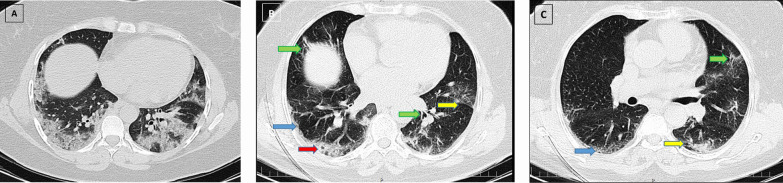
A 47-year-old female patient presented with fever and cough, who was admitted to hospital and was discharged on day 16 of admission. Ground glass opacities and consolidations in multiple pulmonary lobes are observed in the initial CT scan (A). The total CT severity score was calculated as 18, and the disease severity was moderate. Three-month follow-up chest CT (B) shows a parenchymal band (yellow arrow), interlobular septal thickening (blue arrow), mild honeycombing (red arrow), and bronchiectasis (green arrows). Six-month follow-up chest CT (C) shows a few parenchymal bands (yellow arrow) and interlobular septal thickening (blue arrow) and mild bronchiectasis (green arrow), which was diminished in comparison to the previous CT
